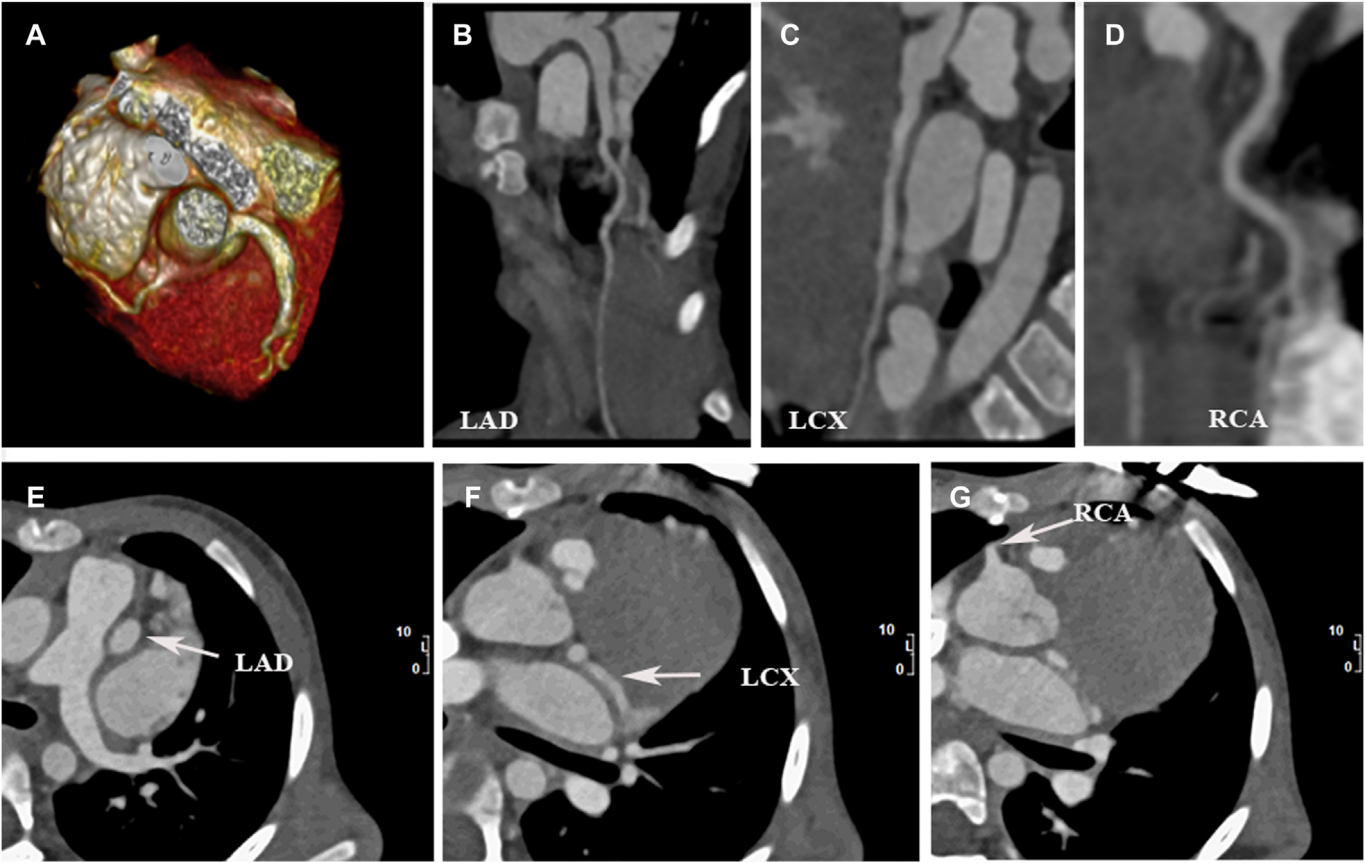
Coronary Computed Tomography Angiography (A) Three-dimensional volume-rendered image shows normal origins of coronary arteries. (B to D) Curved planner reformation demonstrates that coronary artery lumen is unobstructed. (E to G) Axial images show diffuse dilatation of coronary arteries (white arrow), with a maximum diameter of 3.6 mm for the right coronary artery (RCA) and 5.3 mm for the left anterior descending (LAD) artery. LCX = left circumflex artery.
